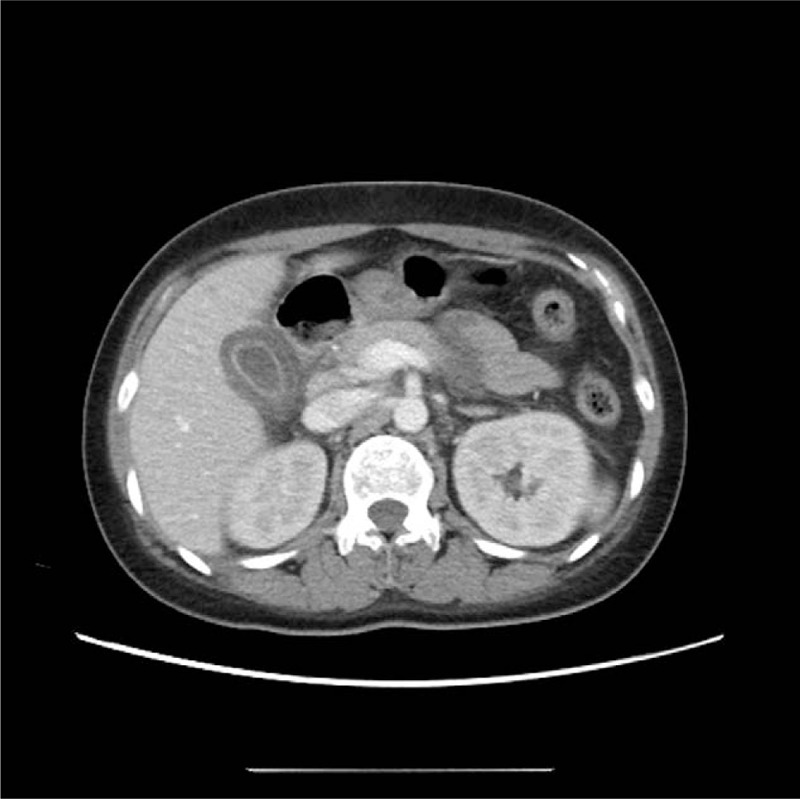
Abdominal computed tomography findings. Edematous gall bladder wall thickening without stones.
